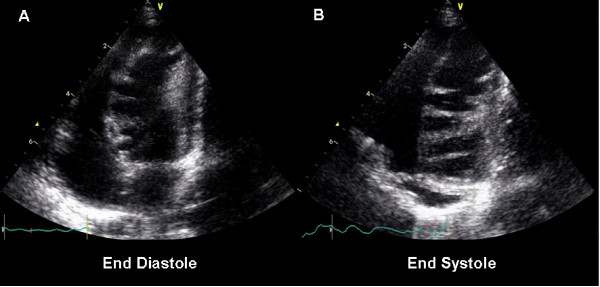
Echocardiogram. Apical 4-chamber view with the transducer angulated towards the inferior LV wall in diastole (A) and systole (B). There are numerous echo dense saw tooth like inwards projections from the inferior interventricular septum and lateral LV wall, clearly seen during both systole and diastole. LV apical dyskinesia was also noted.
